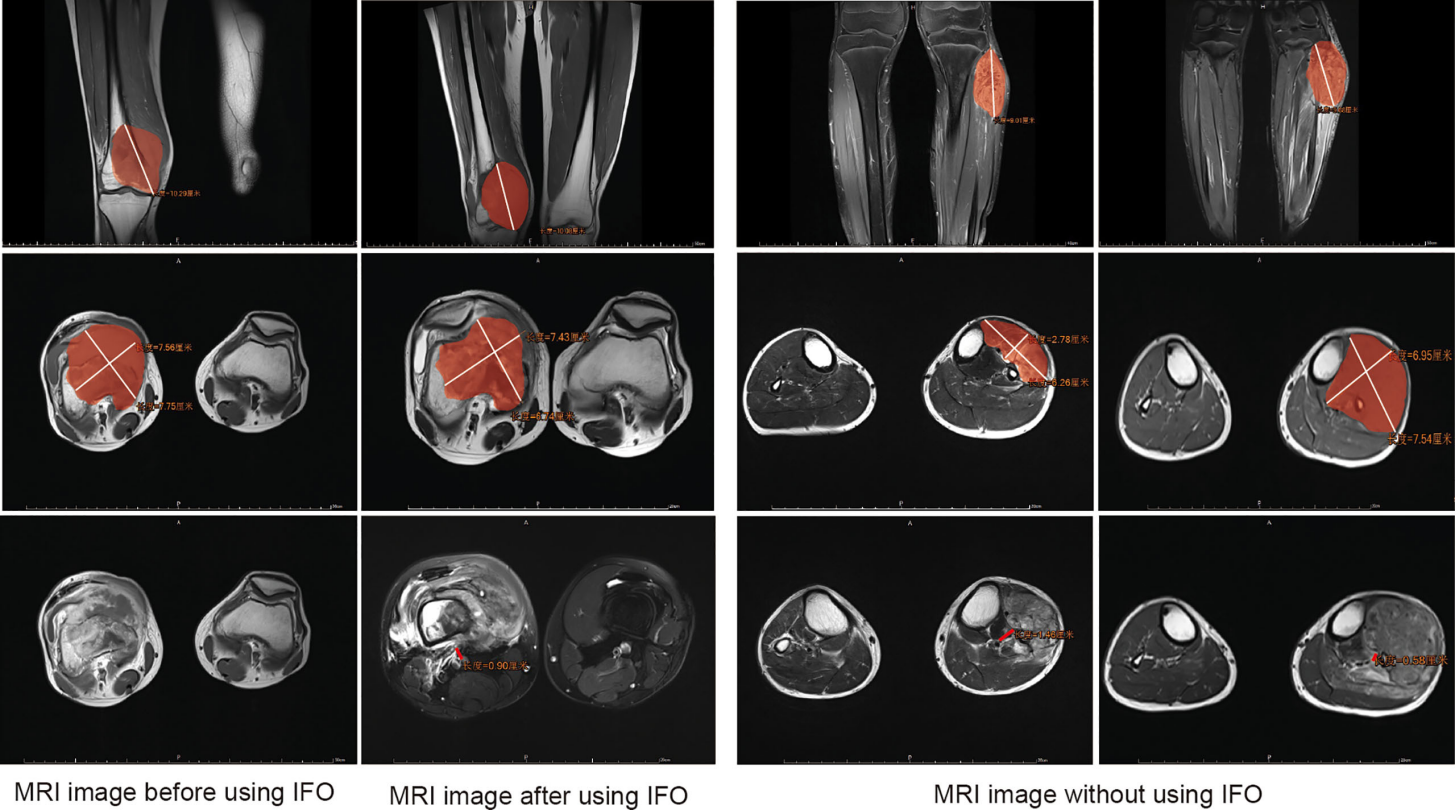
Assessment of the tumor mass volume (TMV) and the shortest distance from the center of main blood vessels to the margin of the tumor lesion (NBT) in MRI. Area covered in red represents the tumor area.
